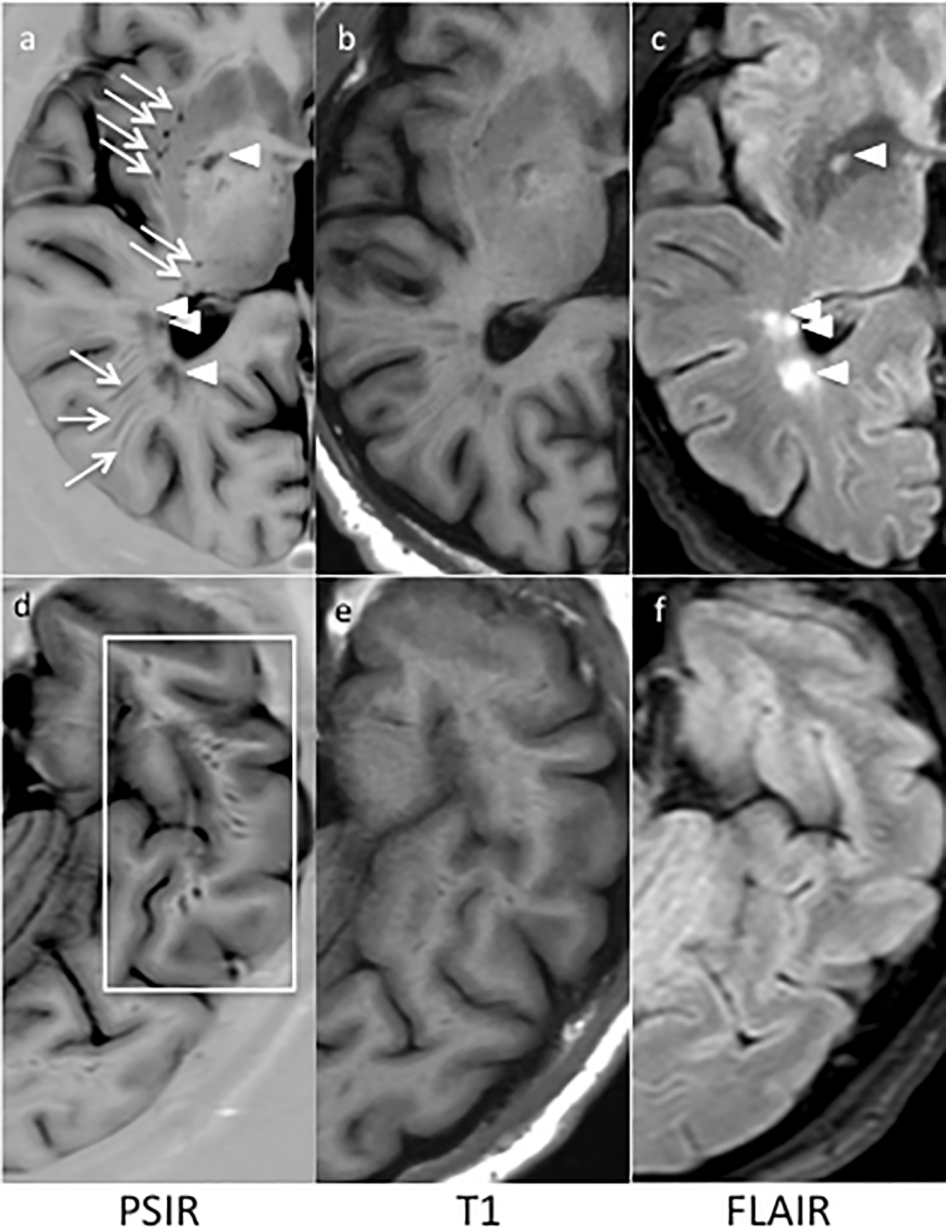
The comparison of PSIR (a,d), T1 (b,e) and FLAIR (c,f) images from a brain of a RRMS patient clearly discloses the superiority of PSIR in detecting eVRS. In (a) eVRS are indicated by arrows while WM lesions are indicated by arrow heads (see the corresponding hyperintense lesions on FLAIR image (c)). In (d), at least 10 eVRS can be counted inside the white rectangular frame, but are hardly identified on the corresponding T1 (e) or FLAIR (f) images. The discrimination between eVRS and MS lesions is easily done by comparison of PSIR and FLAIR sequences.
